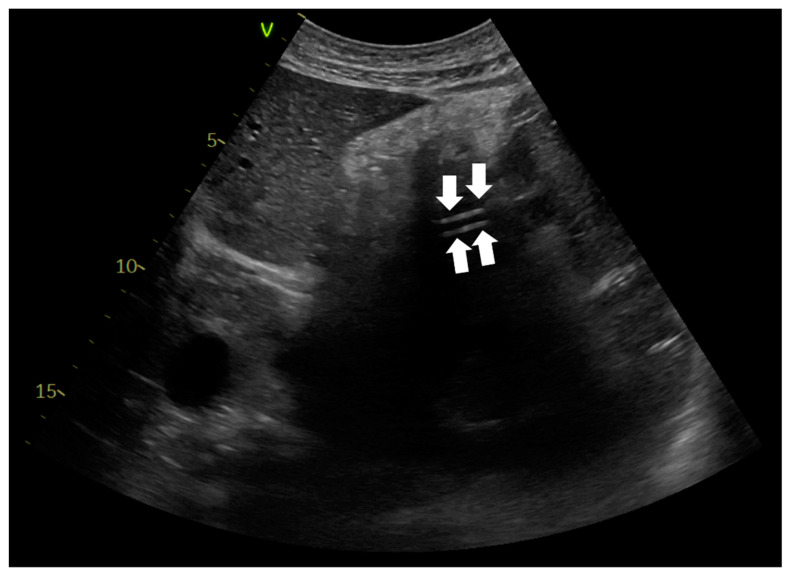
Abdominal ultrasonography. Ultrasonographic confirmation of nasogastric tube presence in the stomach. The liver is seen on the left of the image. Two parallel lines are noted, corresponding to the NGT (white arrows).
